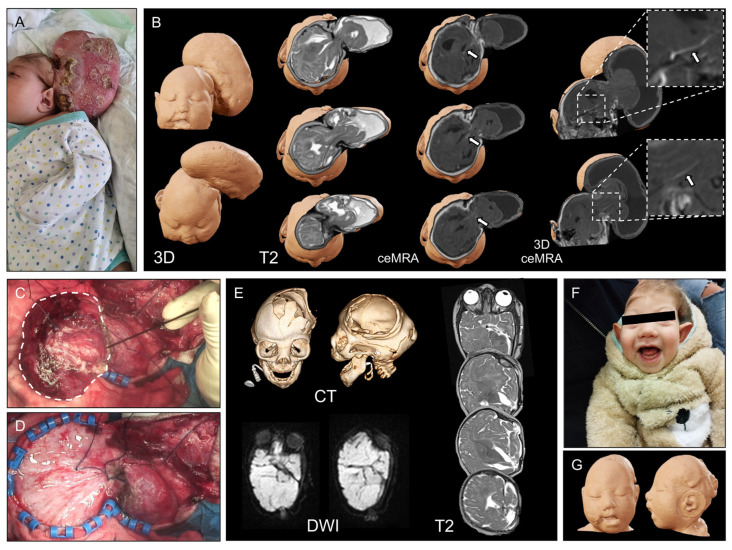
3-month-old with a giant left parietal encephalocele. (A,B) Congenital extrusion of the left supra-insular hemisphere supplied by the dominant M2 branch (arrows). (C,D) Intraoperative situs before (C) and after (D) resection of the herniated parenchyma (dotted line). (E–G) Postoperatively, CT demonstrates barrel stave osteotomy decompression, and MRI shows sufficient neurological and cosmetic outcome without infarcts on DWI.
